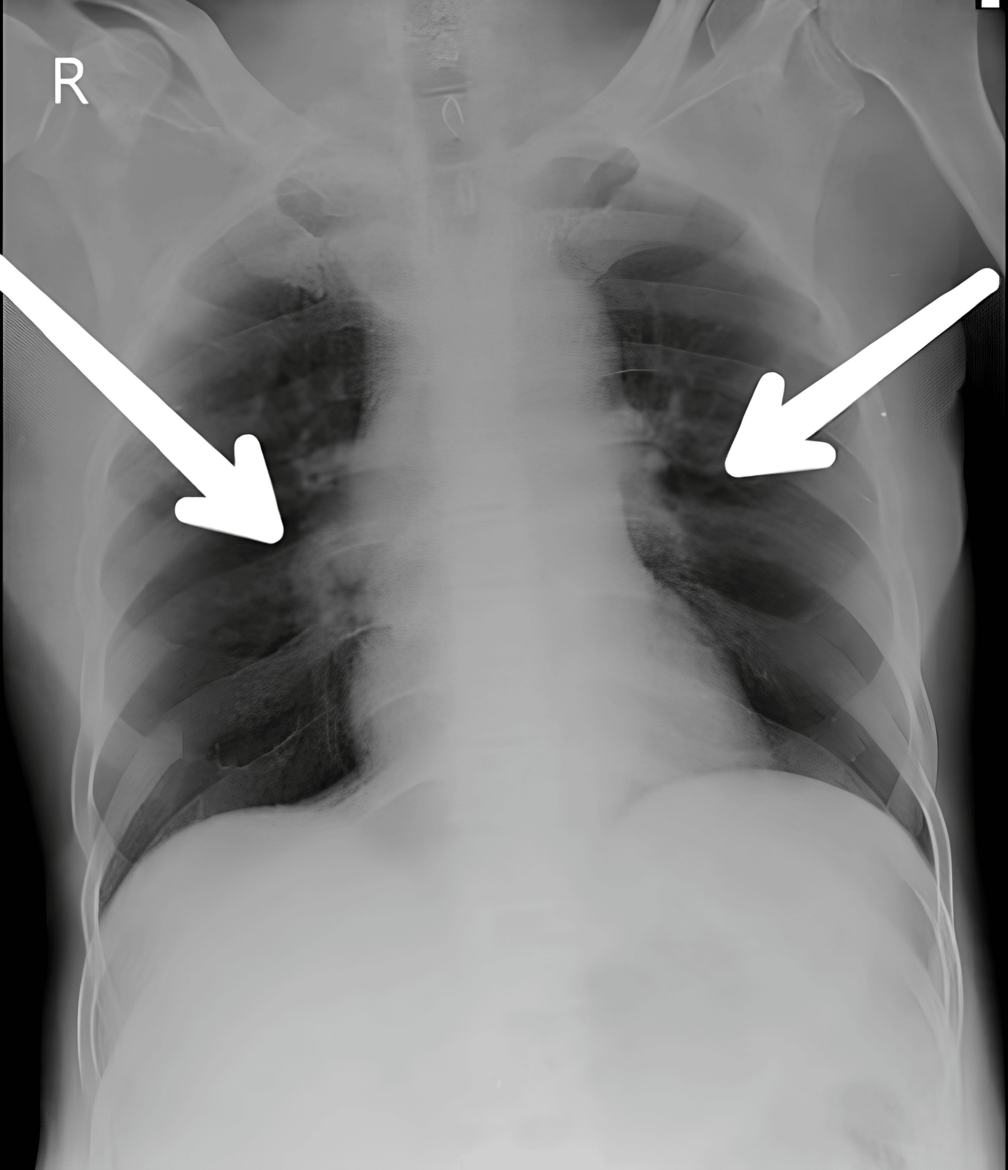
X-ray showing bronchovasicular marking (shown with white arrow)
